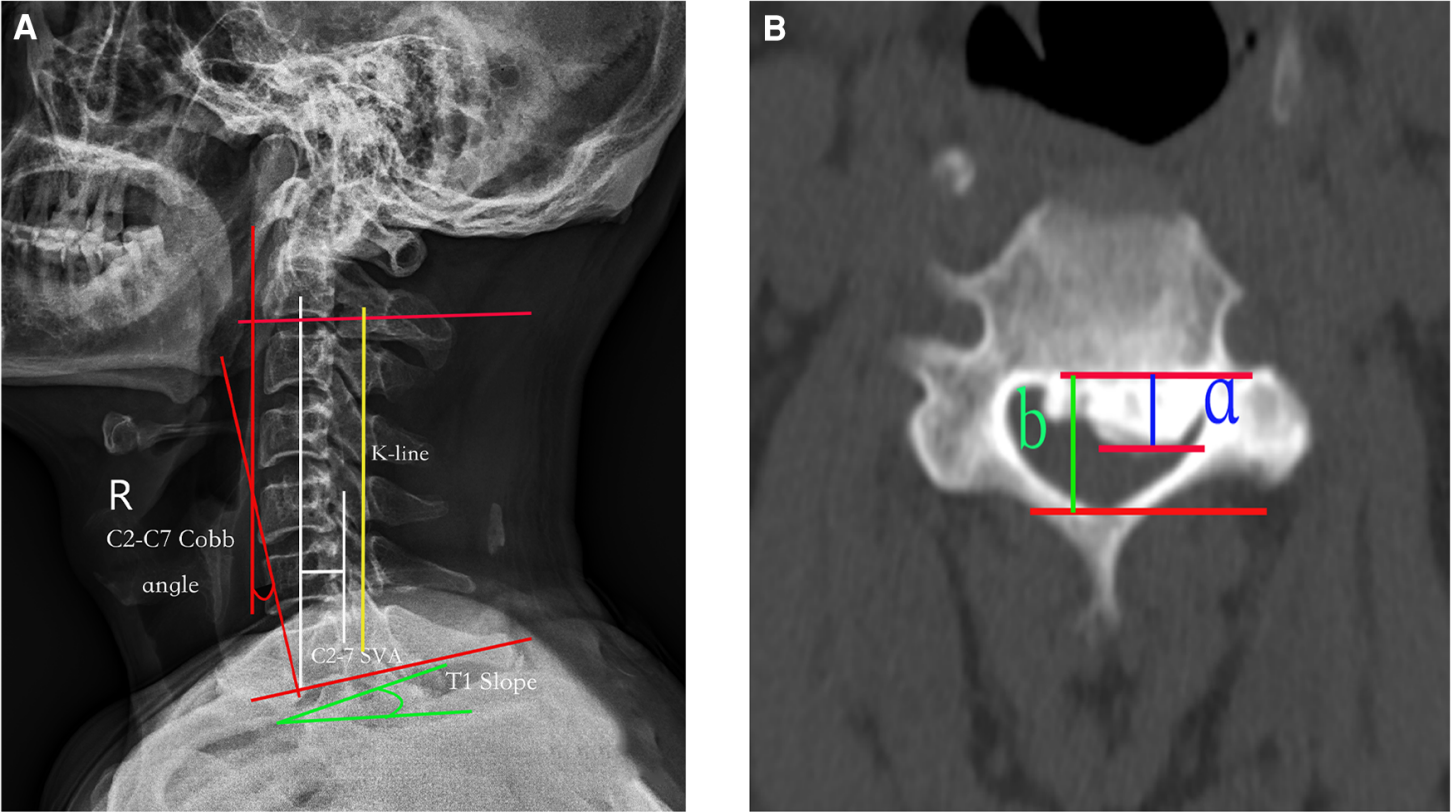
(A) Radiographic measurements: (1) the K-line is a straight line joining the midpoints of the spinal canal at C2 and C7 on a lateral radiograph; (2) C2–C7 SVA: the distance between the C2 plumb line and the superoposterior endplate of C7; (3) C2–C7 Cobb angle: the angle formed by the C2 and C7 lower endplates; and (4) T1 slope: the angle between the horizontal line and the T1 upper endplate. (B) Percentage of spinal canal occupation by ossified mass. The thickness of the ossification block (a, green line) and the anteroposterior diameter of the spinal canal (b, yellow line) were measured on the axial CT section of the highest point of the ossification block. The percentage of spinal canal occupation by the ossification block = a/b × 100%. SVA, sagittal vertical axis.
